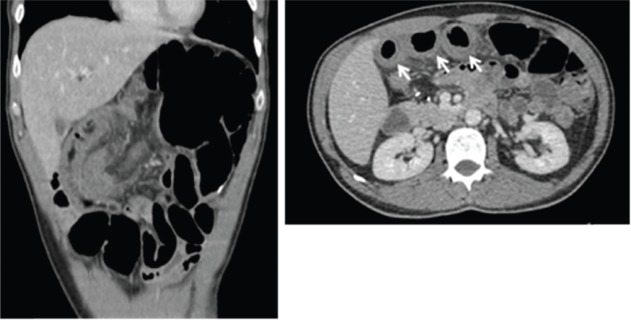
CT scan showing bowel thickening and dilation. Arrow shows small bowel thickening.
